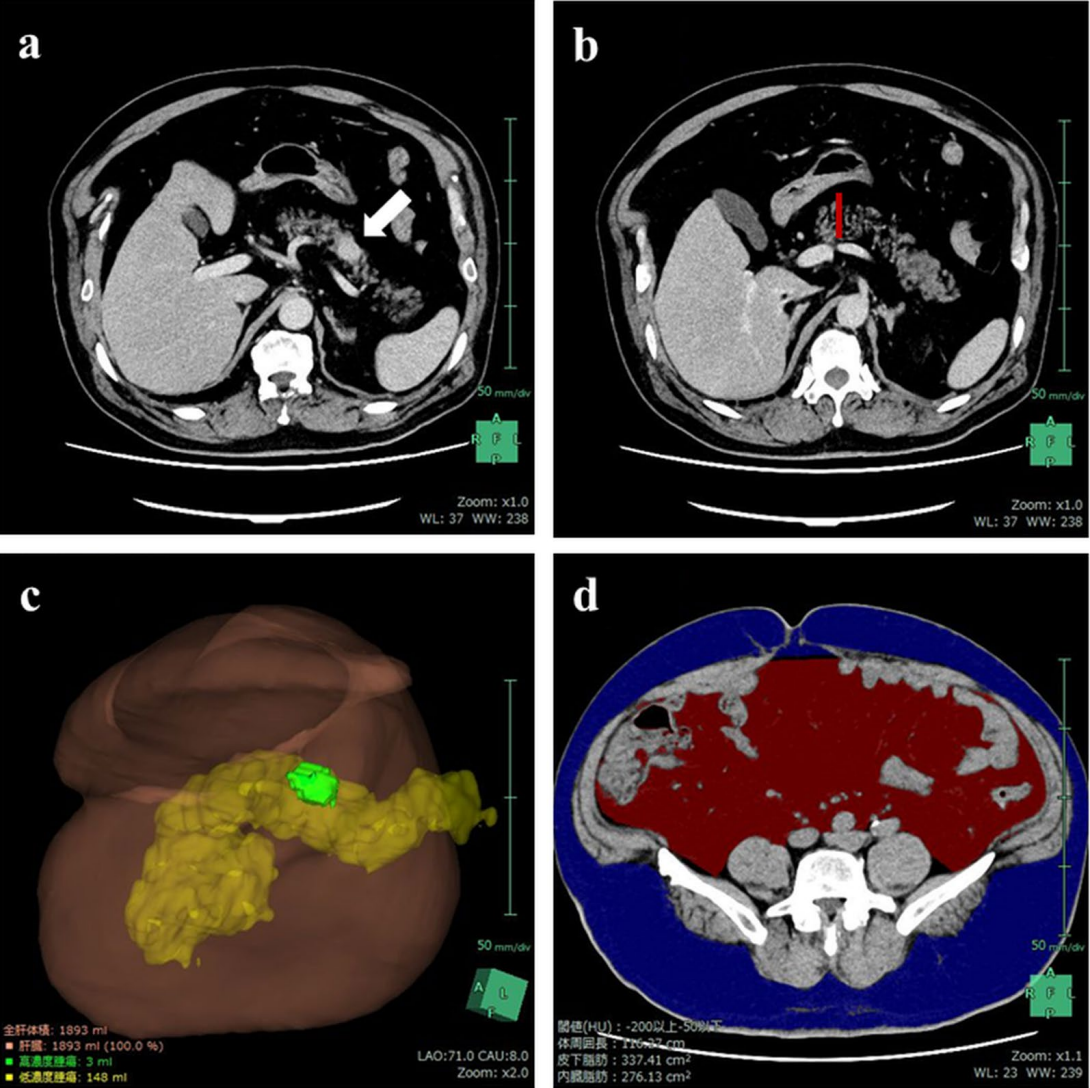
CT scan at initial visit. a Enhanced tumor measuring 15 mm on the pancreatic body (white arrow) and severe fat deposition were observed. b Thickness of the pancreas parenchyma at the bifurcation of the superior mesenteric and the splenic veins was 32 mm (red bar). c CT volumetry revealed that the PV was valuated as 148 ml (yellow structure). d Subcutaneous and visceral fat areas were 337.4 cm^2^, and 276.1 cm^2^, respectively
